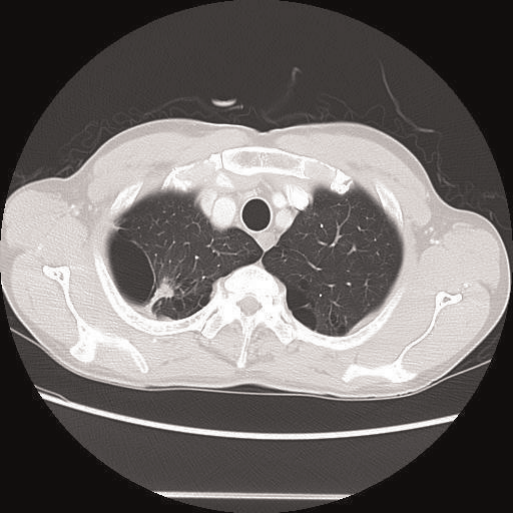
Chest CT. A very small tumor (8 mm) is seen in the right lung apex. The tumor shows irregular contours and invades the pleura.
